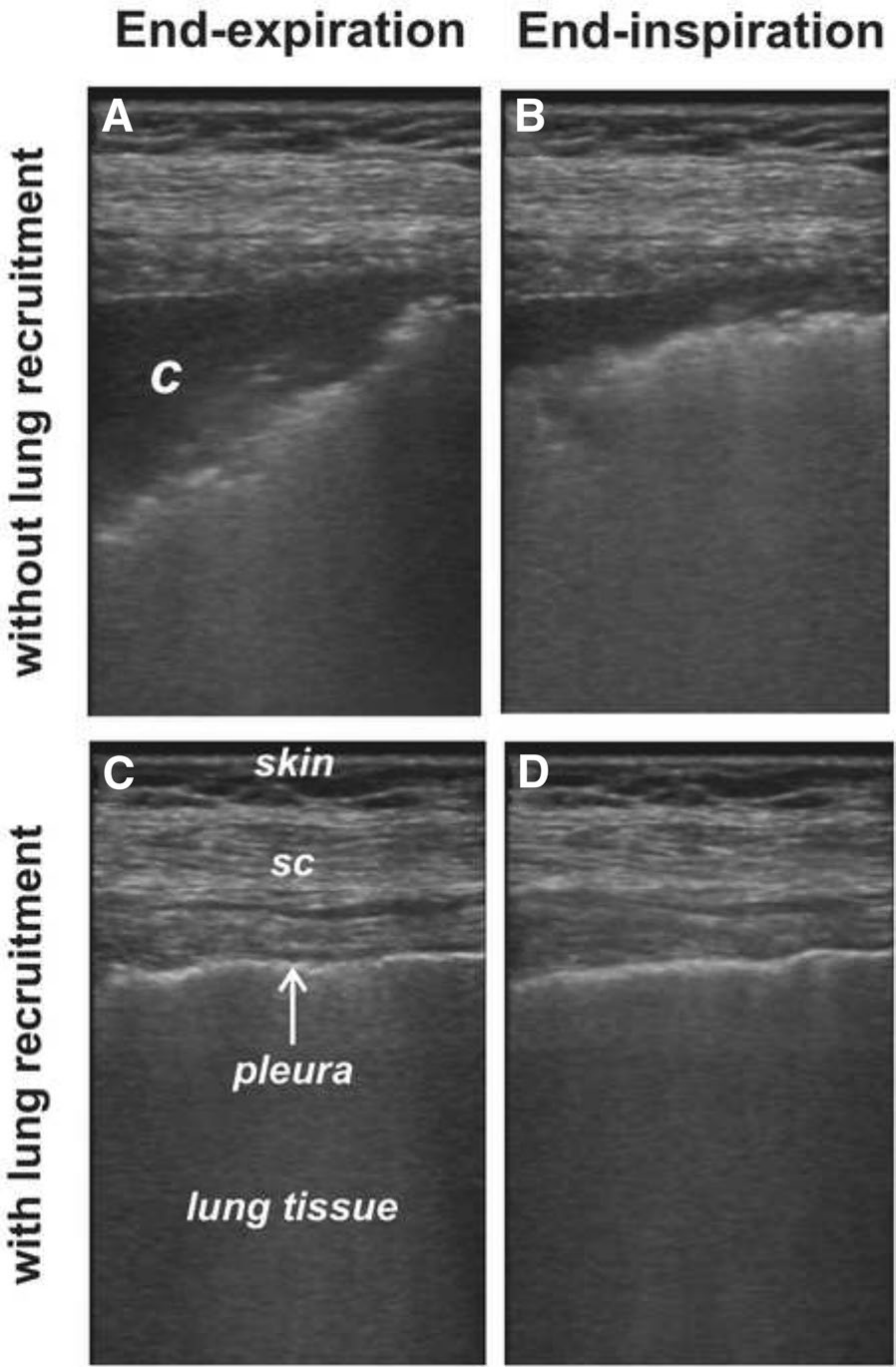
Tidal recruitment in an anesthetized adult. All ultrasound images were obtained at the same lung region. *Figures* show atelectasis and tidal recruitment despite a protective ventilation pattern (A, B). A pulmonary consolidation (*c*) or atelectasis appears as a hypoechoic area mixed with airway bronchograms. Visual differences in the degree of lung aeration and the area of atelectasis between end-expiration and end-inspiration define the presence of tidal recruitment. C and D were obtained after a lung recruitment maneuver. They represent almost normal lung ultrasound images during end-expiration and end-inspiration, with the typical structures of skin, sub-cutaneous tissue (*sc*), pleural line and lung tissue. No differences in aeration were found during the breathing cycle
